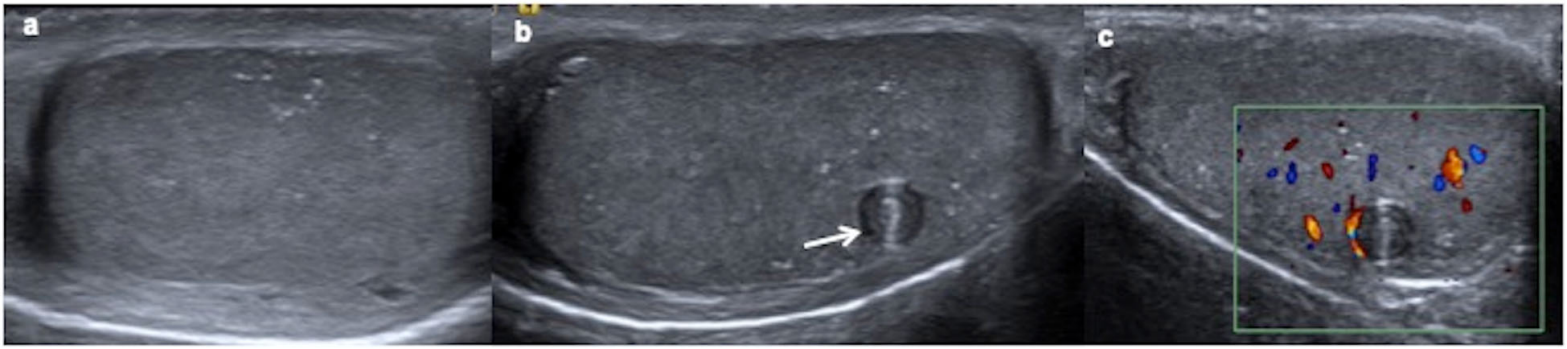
Tumour and microlithiasis. A 12-year-old boy with right scrotal pain. a Longitudinal ultrasound view of the left testicle shows multiple punctuate non-shadowing echogenicities compatible with microlithiasis. b Longitudinal view of the right testicle shows besides microlithiasis, a round well-defined lesion with onion ring appearance (arrow) typically characteristic of epidermoid cyst. c Colour Doppler shows only peripheral vascularization. An intraoperative biopsy diagnoses an epidermoid cyst and a testis sparing surgery is practised
